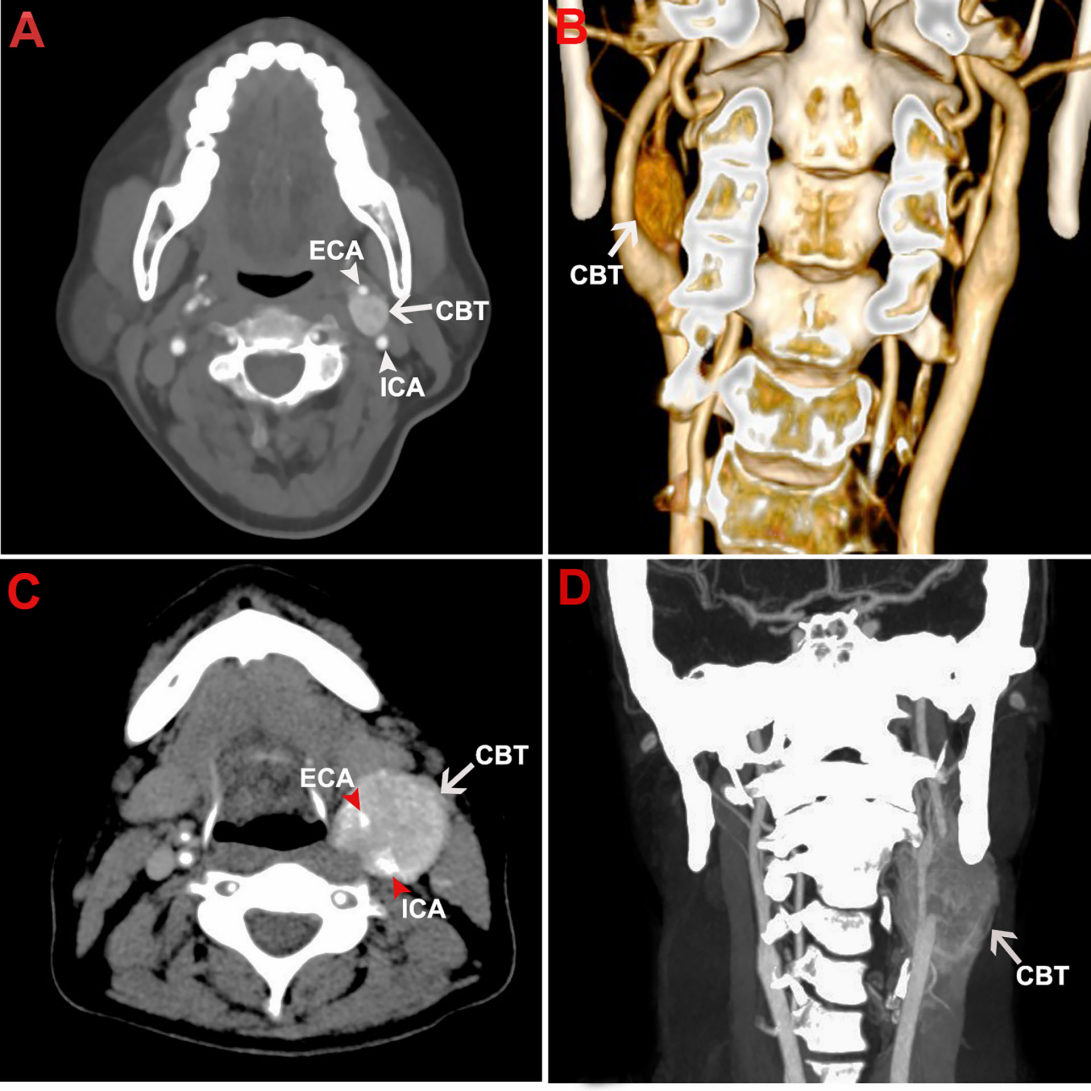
Representative CTA images of CBT. The figure shows the representative CTA images of a Shamblin type I CBT in axial view (A) and coronal view (B). CTA images of a Shamblin type III CBT were shown in axial view (C) and coronal view (D). The tumor lesions displayed typical radiographic characteristics of CBTs with localization at carotid bifurcation, splaying of carotid arteries, and hypervascularity. No encasement and complete encasement to ICA or ECA were noticed in Shamblin I (A, B) and Shamblin III lesions (C, D), respectively. CBT, carotid body tumor; CTA, computed tomography angiography; ICA, internal carotid artery; ECA, external carotid artery.
